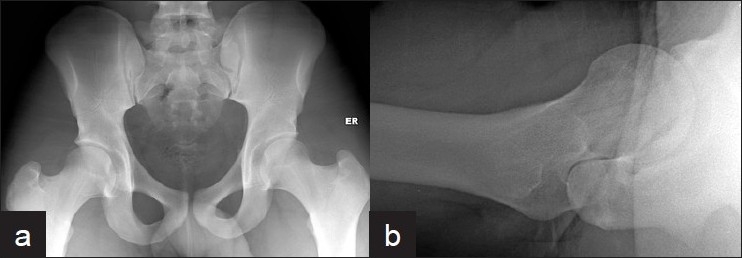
(a) Anteroposterior view of pelvis and (b) cross table lateral view of the right hip several hours after injury showing normal radiograph
